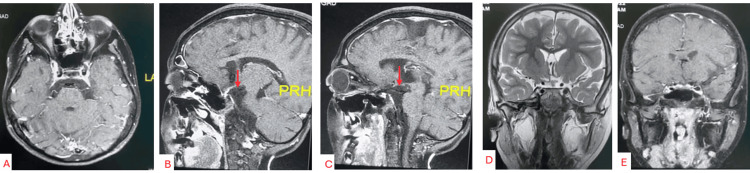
a, b, c, d, e) Axial, sagittal, and coronal planes, postoperative MRI with contrast showing complete tumor resection with intact floor of the third ventricle as exhibited in b, c
